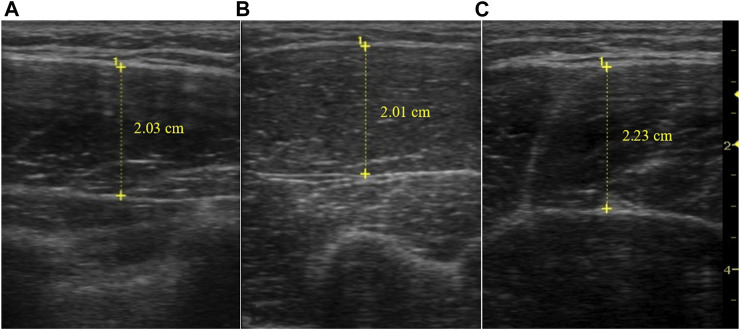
Example ultrasound images of the pectoralis major (A), biceps brachialis (B), and rectus femoris (C) muscle, measurement depth is 5 cm.
